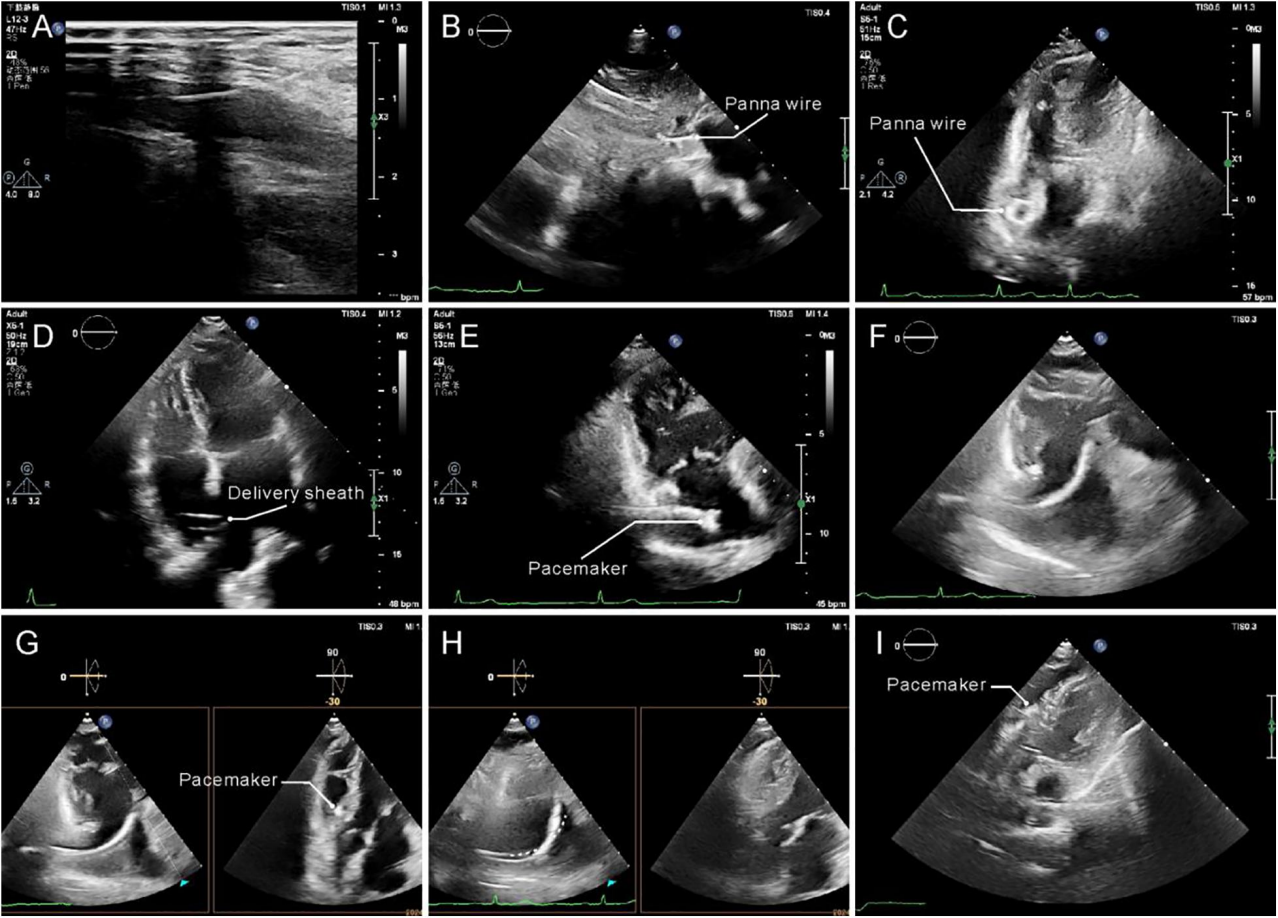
Transthoracic echocardiography guided procedure assisted by Panna wire. (A) Ultrasound guided venipuncture. (B) Subcostal view shows the advance of Panna wire into the RA, the spindle-shaped tip can be easily detected. (C) The Panna wire was delivered to the high right atrium on non-standard trans-apical view. (D) After the insertion of delivery sheath, the Panna wire was withdrawn along with the dilator while the delivery sheath was maintained in the high RA position. (E) The Micra delivery system was inserted through the sheath until it reached the Marker A, which the tip of pacemaker delivery system align with the tip of delivery sheath. (F) The sheath was then withdrawn to Marker B and the Micra system was free to curve across the tricuspid valve. (G) The position of pacemaker was confirmed by trans-apical RV inflow view, where as the biplane view shows the pacemaker attached to the ventricular septum without interaction with the RV free wall. (H) The Micra system was pushed toward the septum to be held at a high tension position (“gooseneck” sign) from its original position (dash line). (I) The pacemaker was deployed after tug test and electrophysiological test. RA, right atrium; RV, right ventricle.
